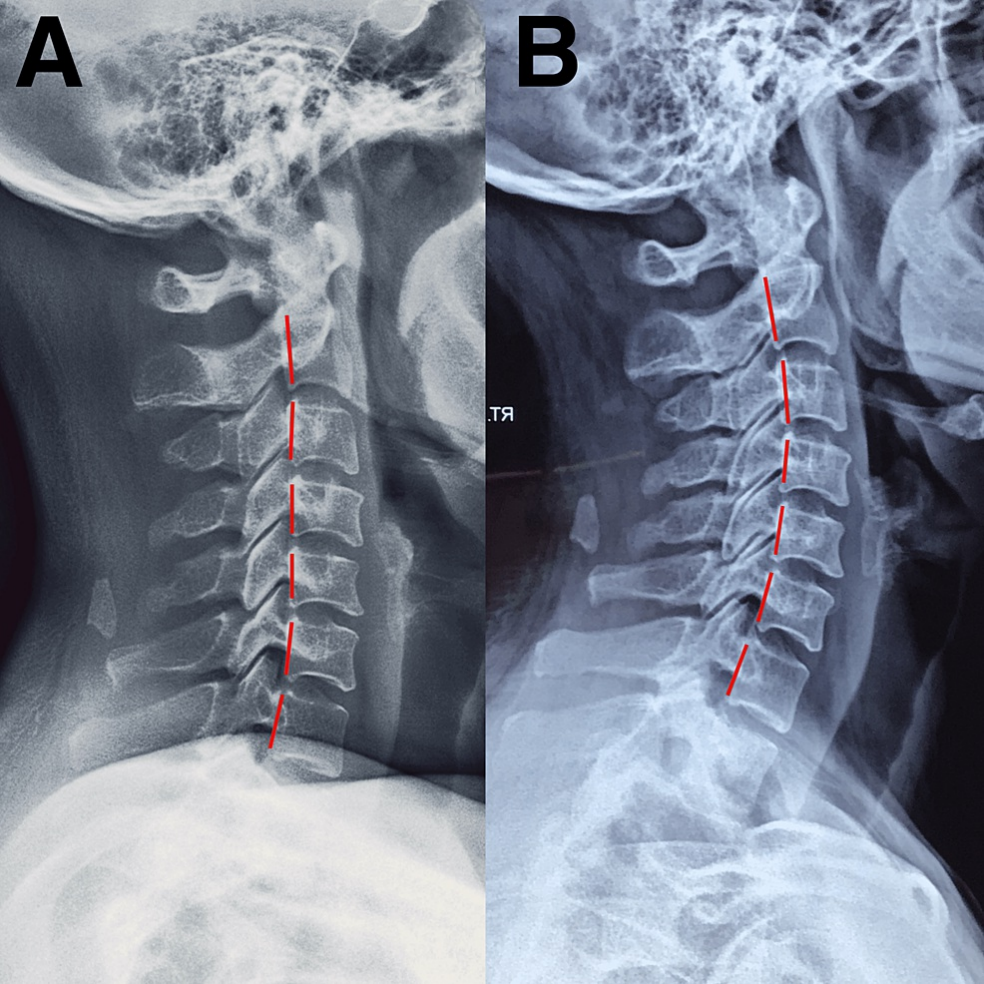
Cervical radiograph in lateral view (A) Cervical alignment demonstrates reduced cervical lordosis. Marginal osteophytes of cervical vertebral bodies and narrowing of C5/C6 disc space were also identified. (B) On the sixth-month re-evaluation, normal bony alignment and articulations were noted.
